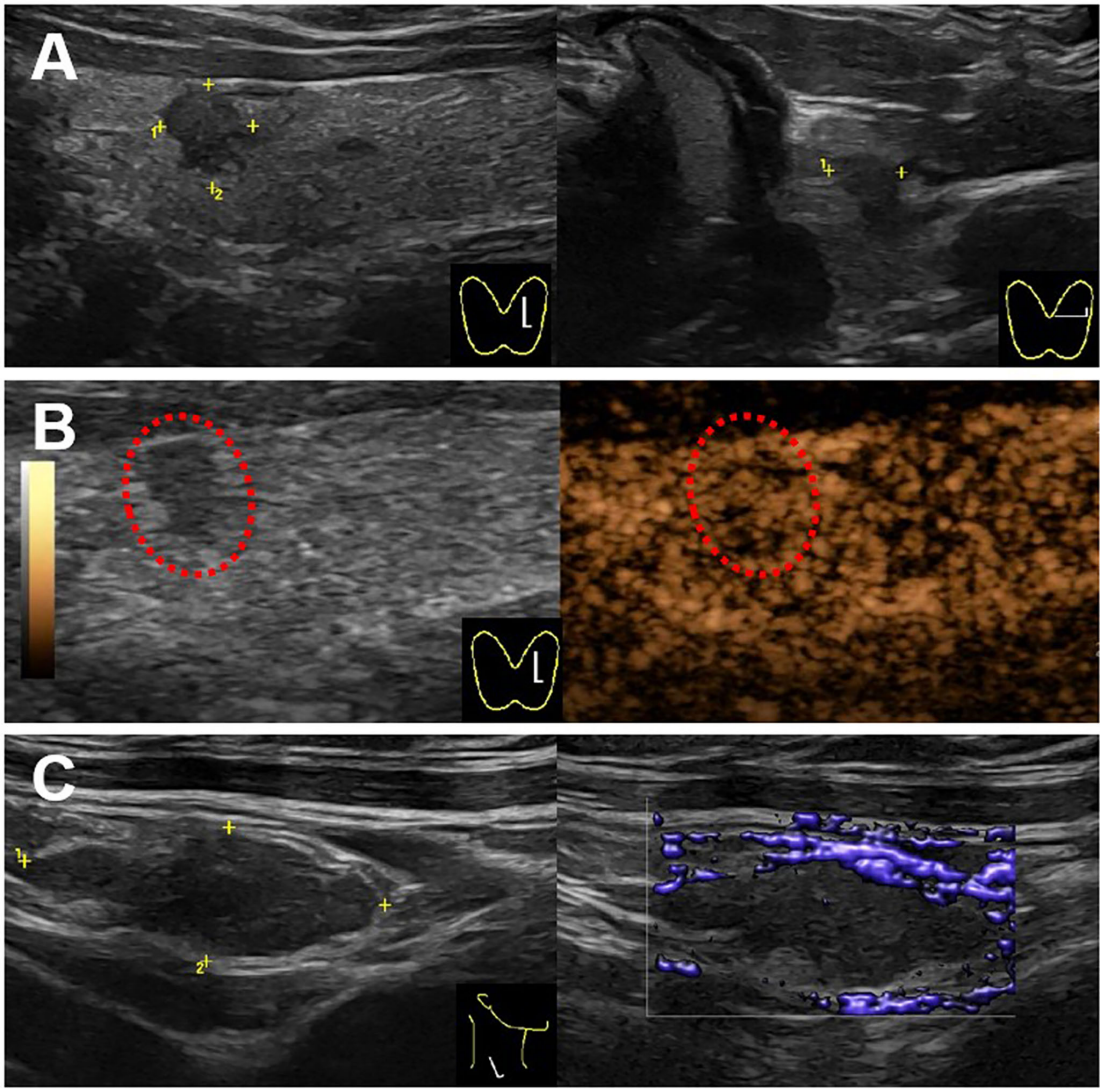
Ultrasound images for the thyroid and cervical lymph node. (A) A hypo-echoic solid nodule with an irregular margin and a taller-than-wide shape (4.7 × 3.7 × 5.3 mm) showed in the upper of left thyroid lobe on the gray-mode ultrasonography. (B) The nodule showed uneven iso-enhancement on the CEUS mode ultrasonography. (C) A swollen lymph node with the absence of fatty hilum (17.4 × 6.6 mm) showed in the right IV compartment of cervical lymph nodes, with no obvious blood flow in the lymph node.
